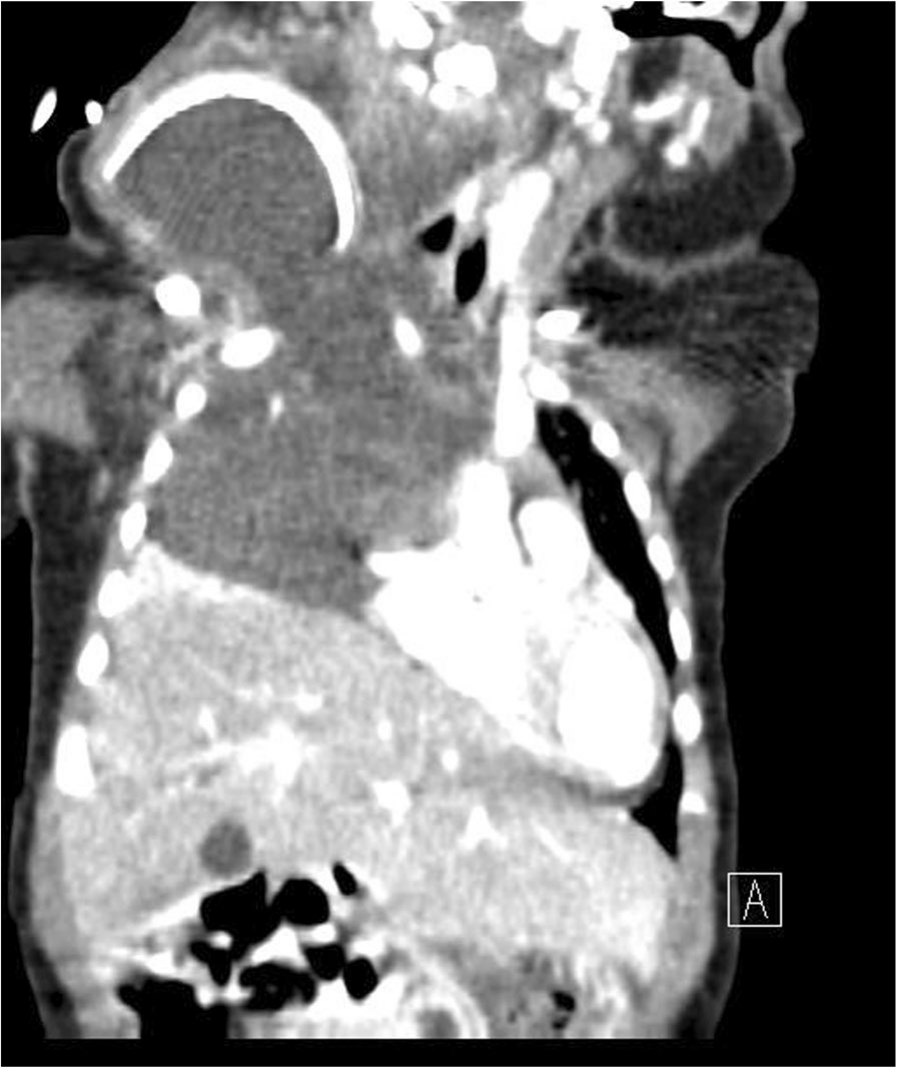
Case 1. Enlarged mediastinal mass after cervical lymphatic malformation excision, visualized on a computed tomography scan
